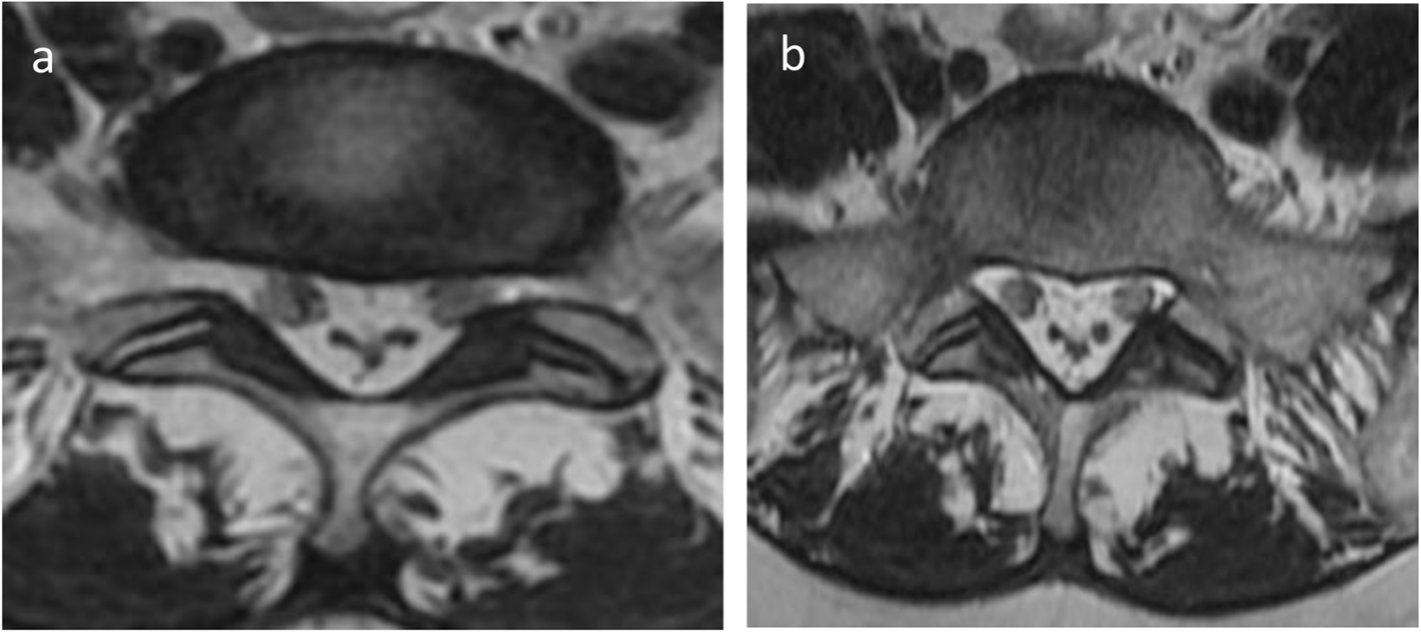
Axial T2-weighted images at L5-S1 interspace (a) and S1 superior end plate (b) demonstrate the pathognomonic “Y” shape of the dural sac
